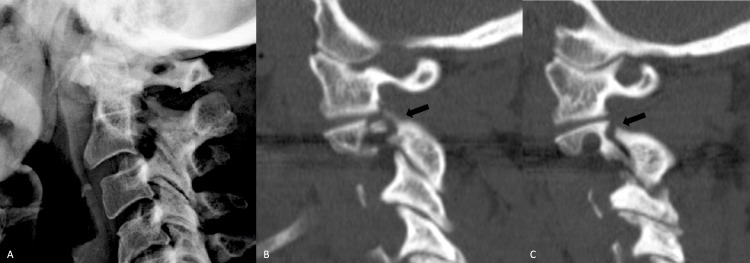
A lateral cervical radiograph (A) two months following the injury shows new anterior displacement of C2 on C3 despite compliance with hard cervical collar immobilization. Sagittal reconstructed paramedian CT images three months following the injury on the right (B) and left (C) show nonunion of the fractures with some widening of the fracture clefts. CT: computed tomography
